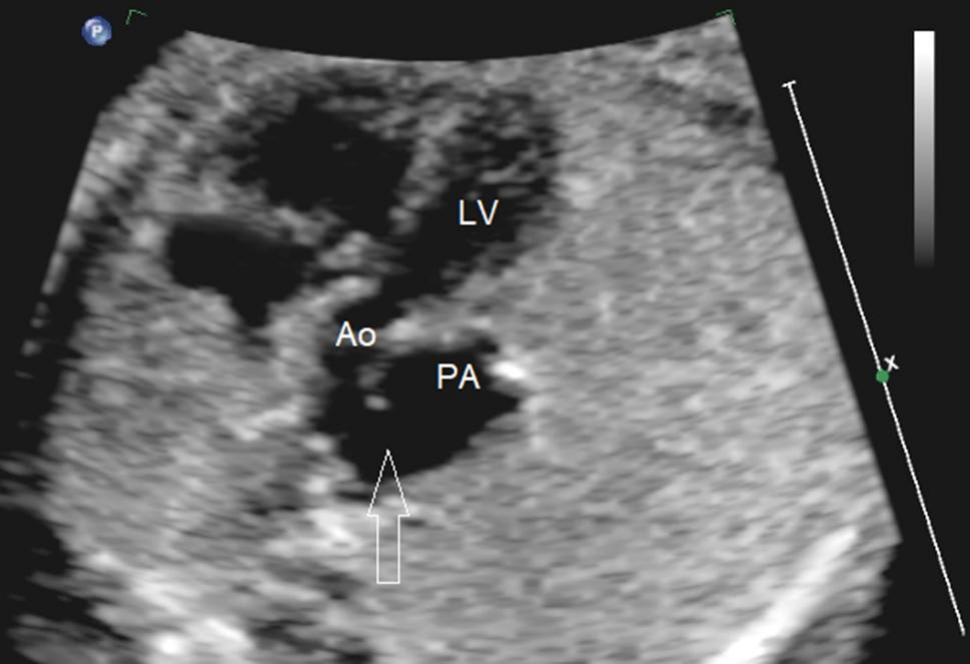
Fetal echocardiographic view showing the left outflow tract and a large AP window (arrow) connecting the aorta with the pulmonary artery. *Ao* aorta, *PA* pulmonary artery, *LV* left ventricle
